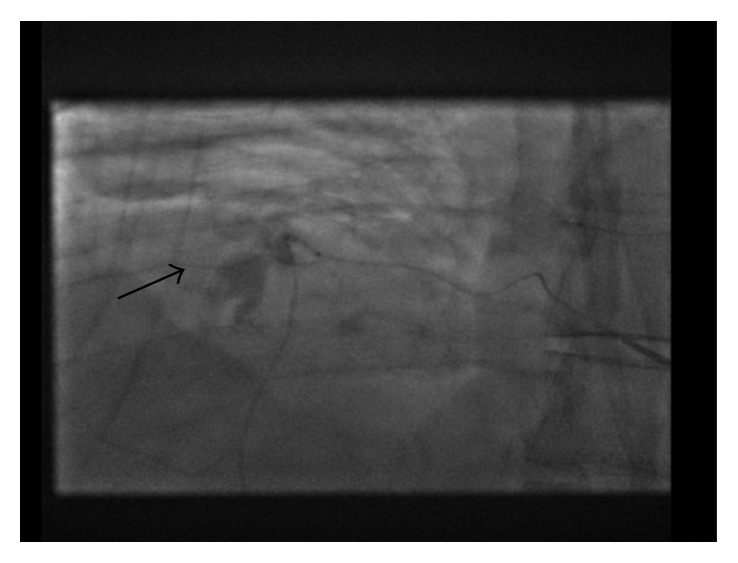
X-ray angiogram of the intercostal arteries shows active contrast extravasation (arrow) from the right T7 intercostal artery.
